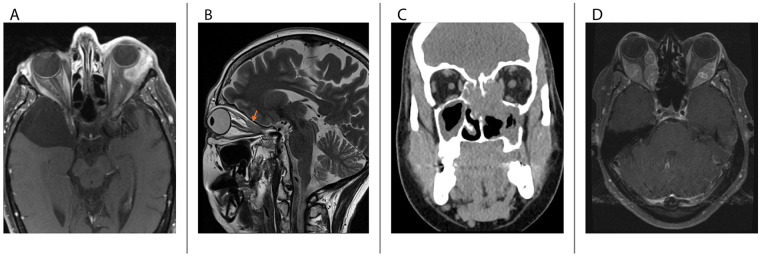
CT and MRI images of primary and secondary orbital tumors. (A) Axial T1 weighted MRI with contrast administration of a carcinoma ex pleomorphic adenoma from the left lacrimal gland. (B) Sagittal T2 weighted MRI of a diffuse large cell B-cell lymphoma in the apex of the right orbit (arrow). (C) Coronal CT scan showing infiltrative growth mainly into the left orbit from a nasal adenocarcioma. (D) Axial, fat suppressed, T1 weighted MRI with contrast administration showing multiple metastases to the extraocular muscles in both orbits from a malignant melanoma of the skin.
